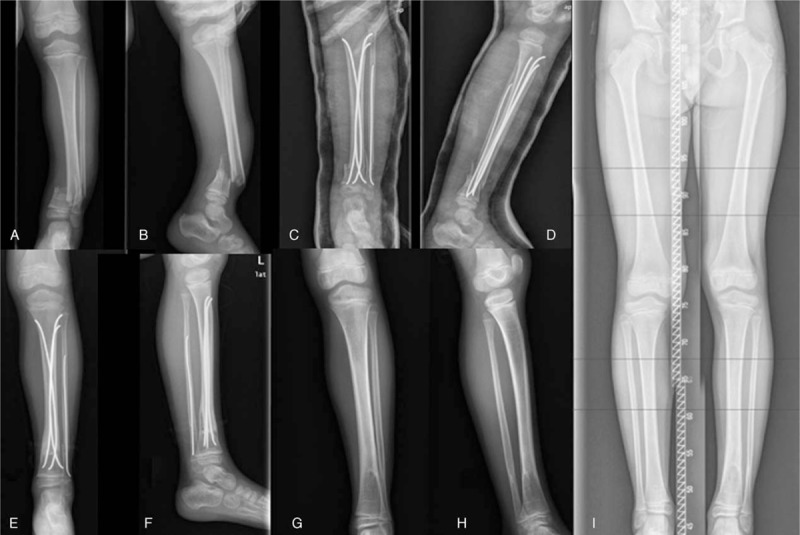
A 5.5-year-old boy suffered traffic accident. A, B, Anteroposterior and lateral views of the severe left DTDMJ fracture, multifragmented open fractures Gustilo type I. C, D, Immediate postoperative radiographs after closed reduction and fixation with ESINs, tibia was fixed with 3 nails, fibula with 1 nail. E, F, 5 months after surgery, the fracture showed radiographic evidence of healing. G–I, 36 months after surgery, no growth arrest or disturbances by radiological assessment, standing weight-bearing view showed 11 mm overgrowth on the left leg. DTDMJ = distal tibial diaphyseal metaphyseal junction, ESIN = elastic stable intramedullary nailing.
